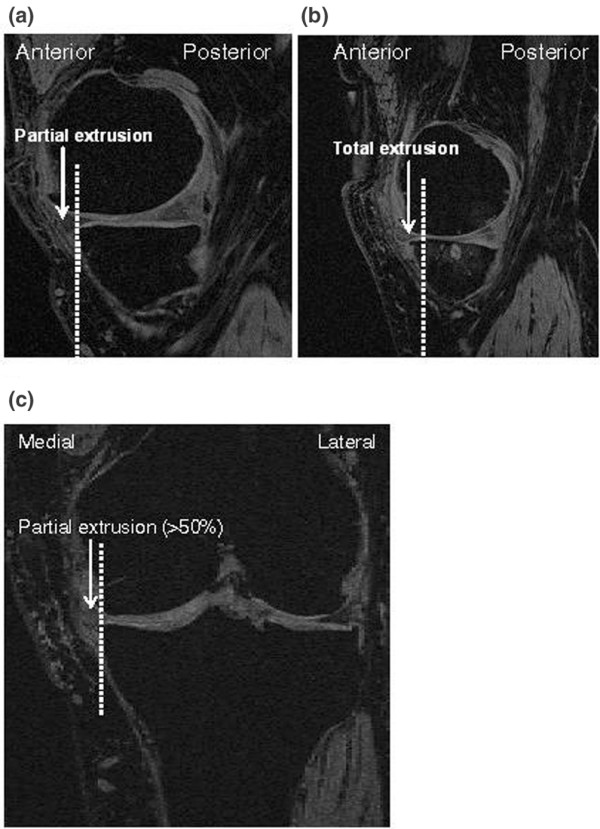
Representations of meniscal extrusion as seen using magnetic resonance imaging. Human knee medial compartment: (a) and (b) sagittal views, and (c) reconstructed coronal view. Partial and total meniscal extrusion at the anterior horn are shown in panels a and b, respectively. Panel c shows a partial (> 50%) meniscal extrusion at the body site.
